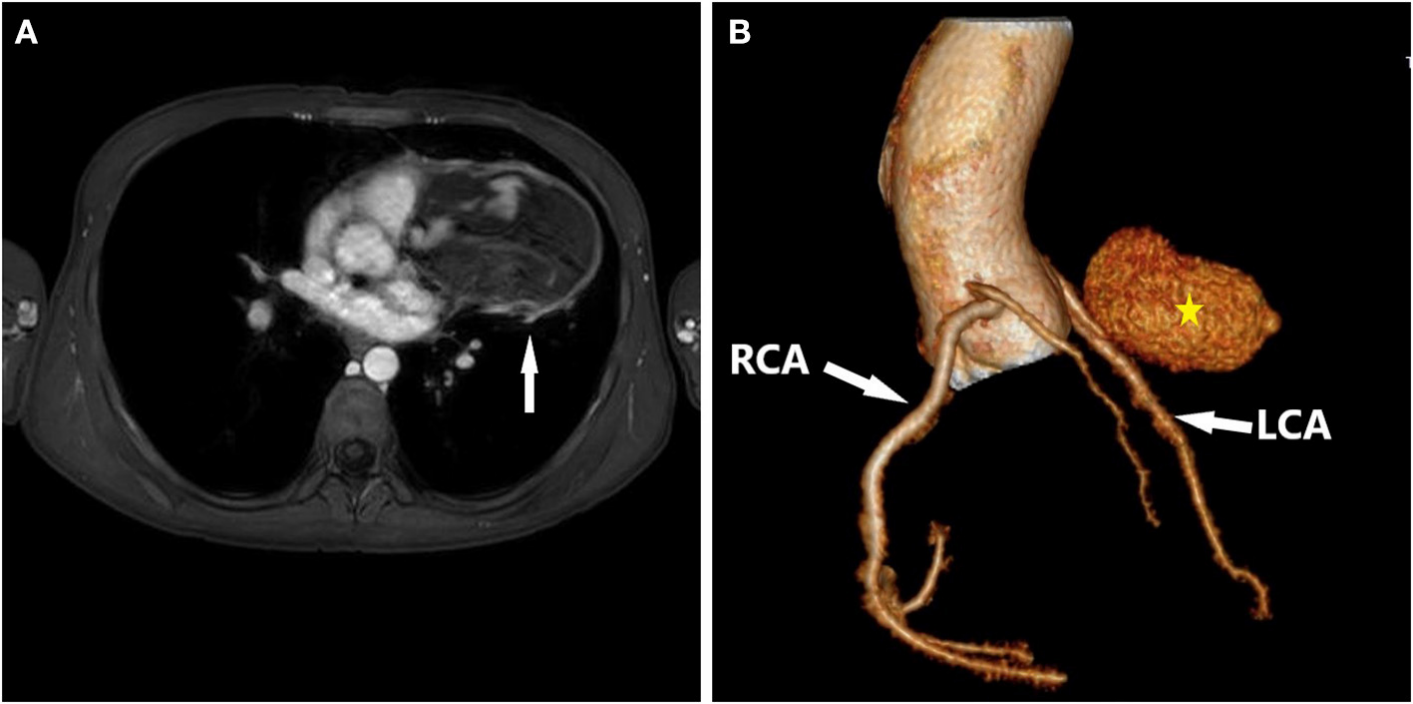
Preoperative chest MRI and Postoperative cardiac CTA. (A) MRI showed a large mixed-signal shadow in the left side of the middle mediastinum, measuring about 9.8 cm × 8.0 cm, with multiple patchy T1W1 high-signal shadows within it, and enhanced scans showed nodular enhancement in the lesion, measuring about 2.6 cm × 1.7 cm. (B) Preoperative cardiac CTA showed the middle segment of the anterior descending branch of the left coronary artery was poorly displayed, and a localized sac-like enhancement shadow was seen, with a maximum cross-section of about 2.6 cm × 1.7 cm.
